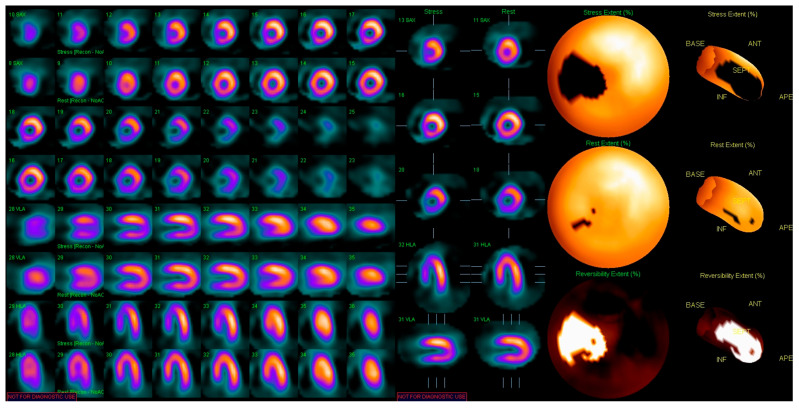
Myocardial perfusion SPECT imaging post-stress and at rest in a 76-year-old female patient with old anterior myocardial infarction and chronic LAD occlusion at coronarography: effort up to 95W, maximum HR of 145 bpm (99% of maximal HR), maximum BP 200/100 mmHg, no angina; resting BP 150/70 mmHg, resting HR 85 bpm. SPECT scan shows a reversible hypoperfusion area at the level of the interventricular septum, especially in the anterior territory, with a differential perfusion score of 11%. SPECT, single photon emission computed tomography; LAD, left anterior descending artery; HR, heart rate; BP, blood pressure.
